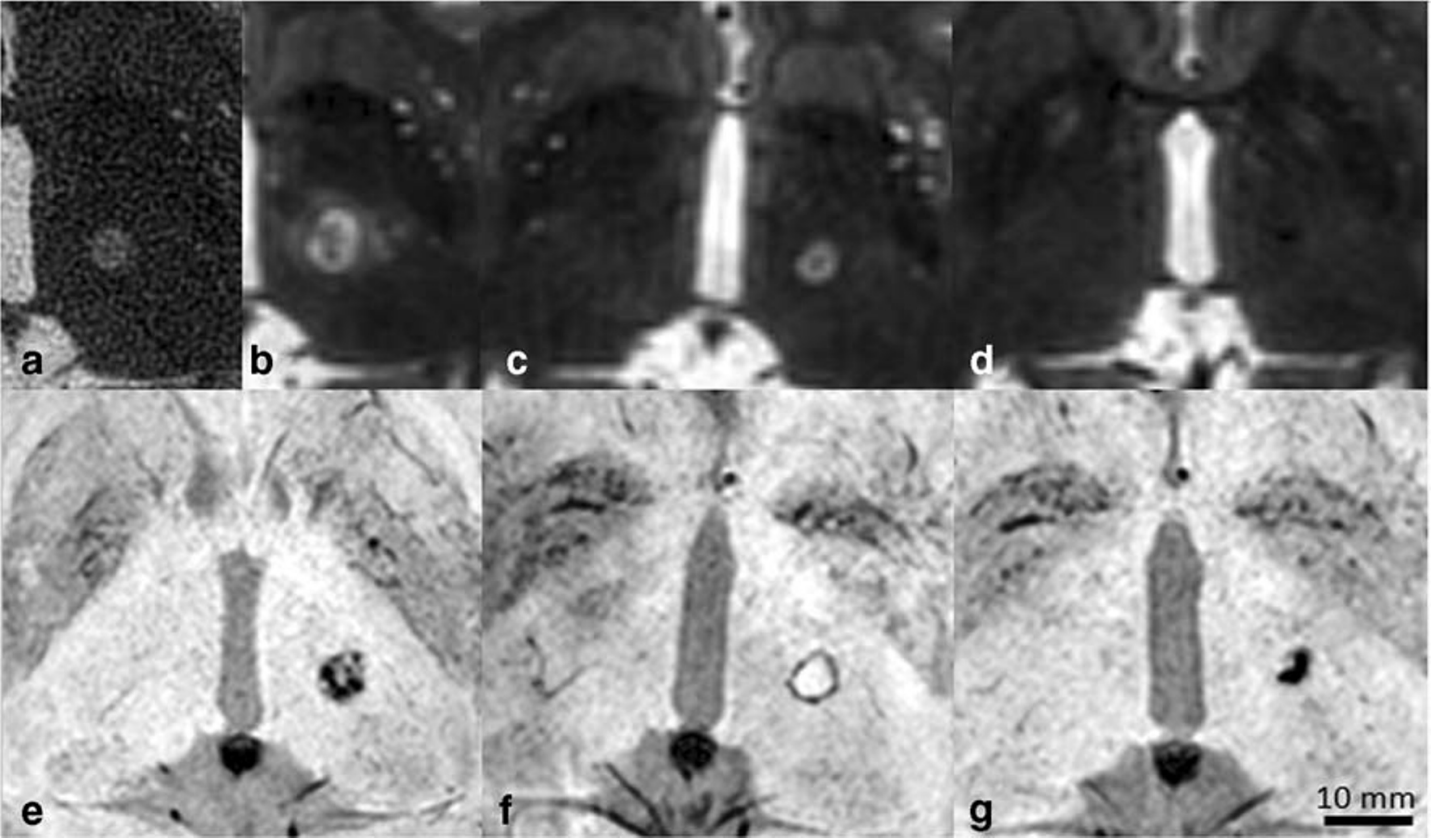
Lesion dynamics at 3-T on T2-weighted and susceptibility-weighted images over time. a–d T2-weighted images and e–g susceptibility-weighted images. a Thirty minutes after therapy, b 3 days, c 30 days and d 180 days after therapy. e Three days, f 30 days and g 180 days after therapy. Note how the lesion first increases in size during the first 3 days (a vs. b), while it has completely vanished half a year after therapy on T2-weighted images and yet remains distinctly visible on susceptibility-weighted images. Image b also depicts the oedema surrounding the core lesion separated by a fine hypointense dark rim. The noisy aspect of image a is due to the distant MRI-integrated body coil used, while the patient was still wearing the treatment helmet
